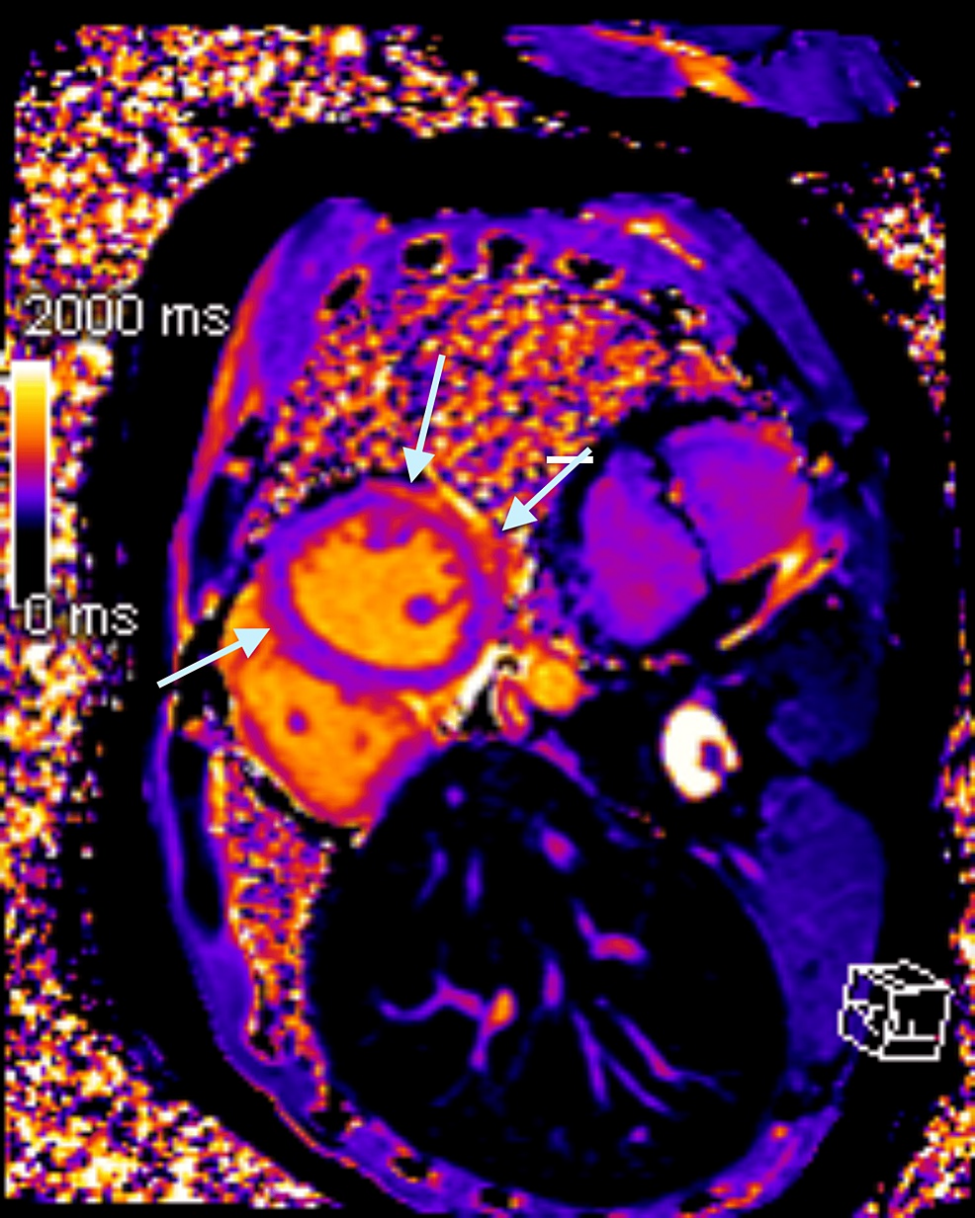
Cardiac MRI demonstrates the prolongation of T1 relaxation time in the subepicardial anterolateral and posterolateral walls, consistent with myocardial edema. Short-axis cardiac MRI performed at 1.5 T shows myocarditis. The native T1 map demonstrates prolongation of the T1 (1250 -1350 msec) relaxation time in the subepicardial anterolateral and posterolateral walls (arrows), consistent with myocardial edema.
